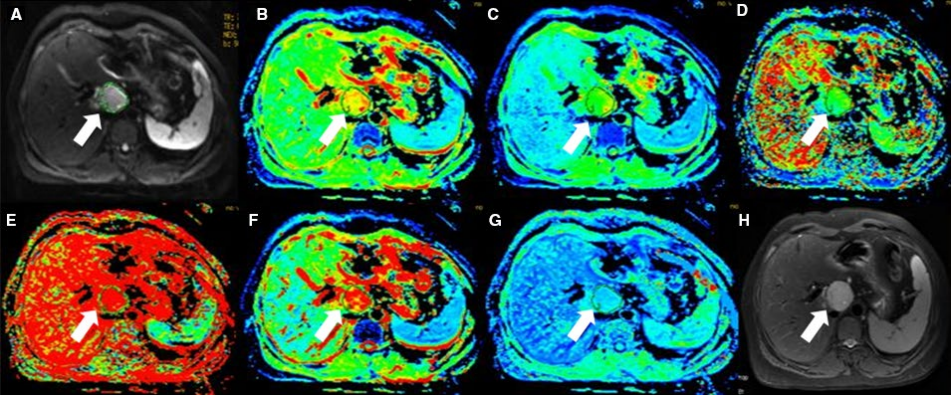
Forty‐five‐year‐old female with hepatic hemangiomas (arrow). (A) is b value of 50 s/mm^2^ of DWI, and (B‐G) are pseudocolor of ADC,* D*
